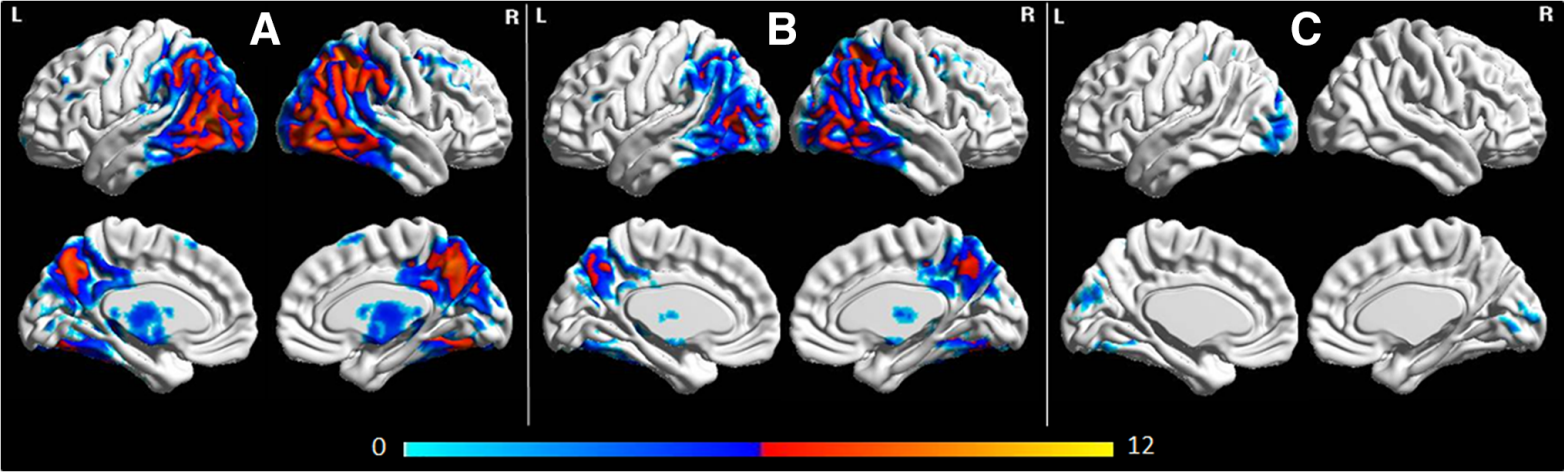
Three-dimensional brain renderings showing FDG-PET hypometabolism in the entire PCA cohort compared to controls (a), the early PCA subjects compared to controls (b), and early PCA compared to the rest of the PCA patients (c). Renders were generated using the BrainNet Viewer (http://www.nitrc.org/projects/bnv/)
